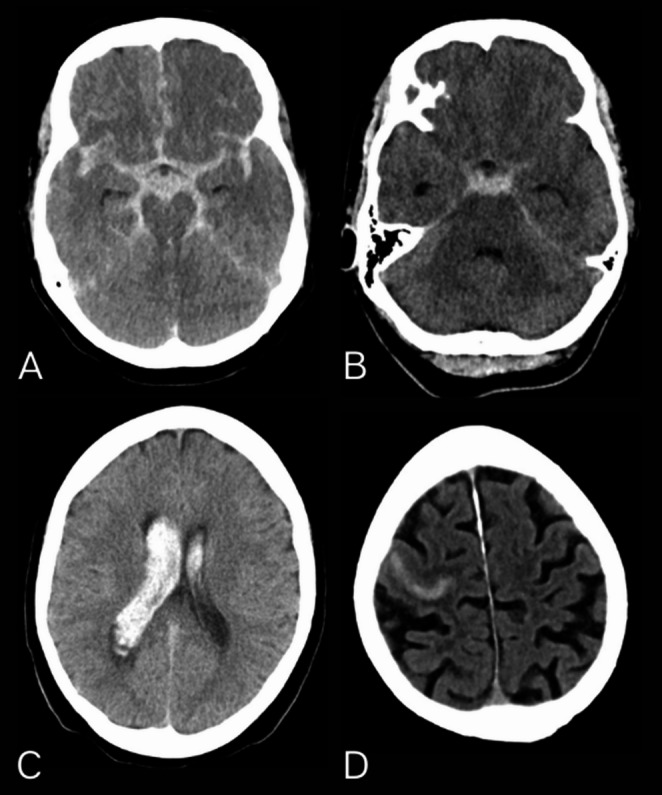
Non-contrast cranial CT examples of subarachnoid hemorrhage. A. Diffuse SAH: Hemorrhage is observed within the interhemispheric fissure, extending into both lateral Sylvian fissures B. Perimesencephalic SAH: Blood is concentrated in the interpeduncular cistern and extends into the basal Sylvian fissure C. Isolated intraventricular SAH: Hemorrhage is confined to both lateral ventricles, with no blood present in the subarachnoid space or other intracranial regions D. Sulcal SAH: Blood is localized to the right precentral sulcus, demonstrating a localized sulcal hemorrhage
